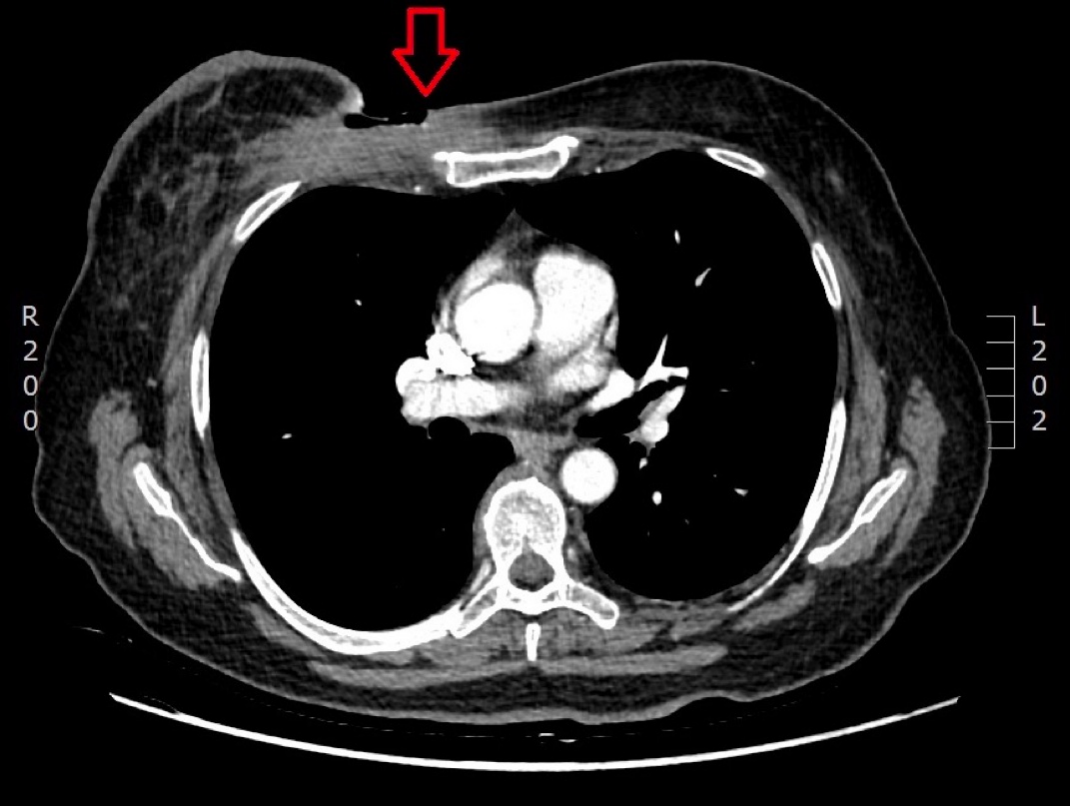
CT scan after two cycles of chemotherapy The primary bulky tumor has significantly reduced in size (*arrow) *
